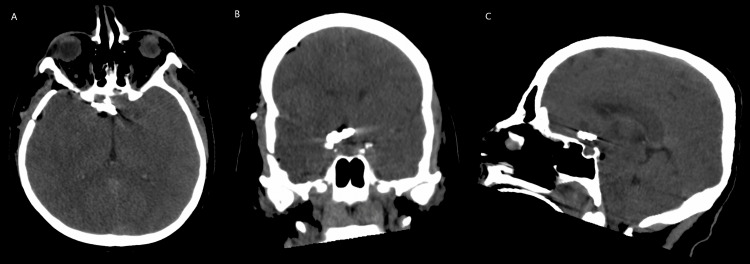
Post-operative non-contrast computed tomography of the head Axial (A), coronal (B), and sagittal (C) views were obtained identifying appropriate clipping of the anterior communicating artery aneurysm without new hemorrhage.
